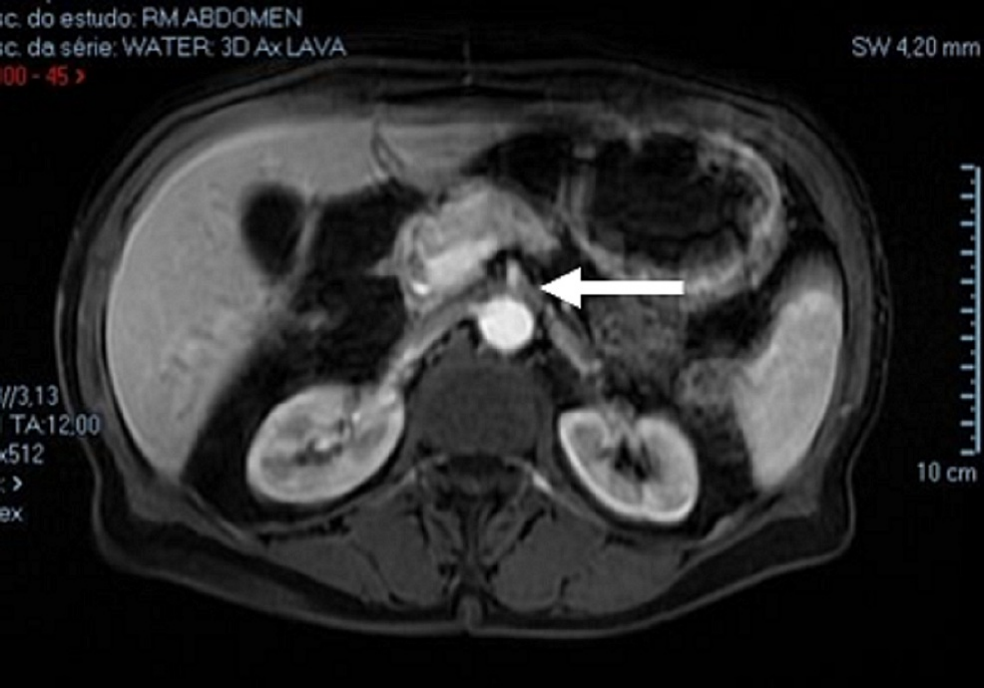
Abdominal MRI Considerable gastric and proximal duodenum distension until the aortomesenteric angle with collapsed distal duodenum. White arrow signalizing the aortomesenteric angle.
